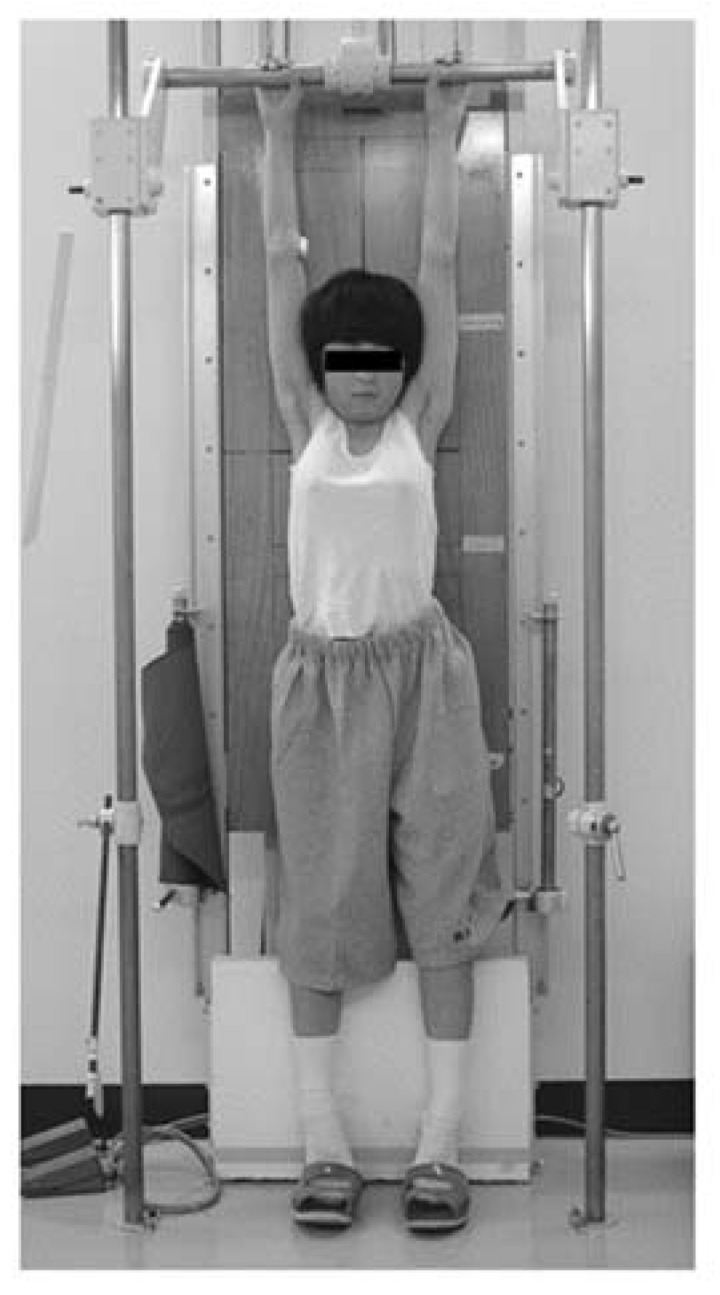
Position of hanging total spine X-ray. Hanging total spine X-ray was taken in a position that the patient is hanging onto the bar, stretching the back, and touching the toes lightly to the floor, not to sway the body under the instruction of making a great effort to stretch their back as much as possible [16] (licensed under CC BY).
